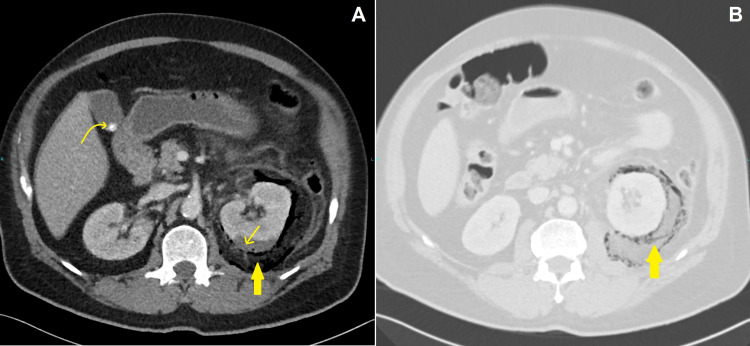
Enhanced abdominal CT axial slices in soft tissue window (A) and lung window (B) of the mid renal poles during the nephrogenic phase A well-organized subcapsular mixed gas-fluid collection in the posterior aspect of the left renal pole is seen (Thin arrow) associated with ipsilateral perinephric gas densities (Thick arrow) comparable to the intraluminal colonic and extracorporeal gas, with thickening of Gerota's fascia seen in (A). It is noteworthy to acknowledge the good renal parenchymal enhancement in the nephrogenic phase. Note the incidental finding of an uncomplicated calcified gallstone (Curved arrow).
